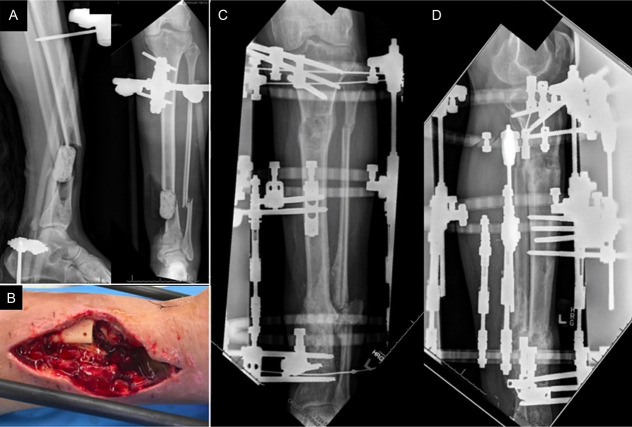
Radiograph (A) and clinical image (B) after initial debridement and removal of intramedullary nail. Radiographs after revision debridement followed by distraction osteogenesis/bone transport (C, D).
